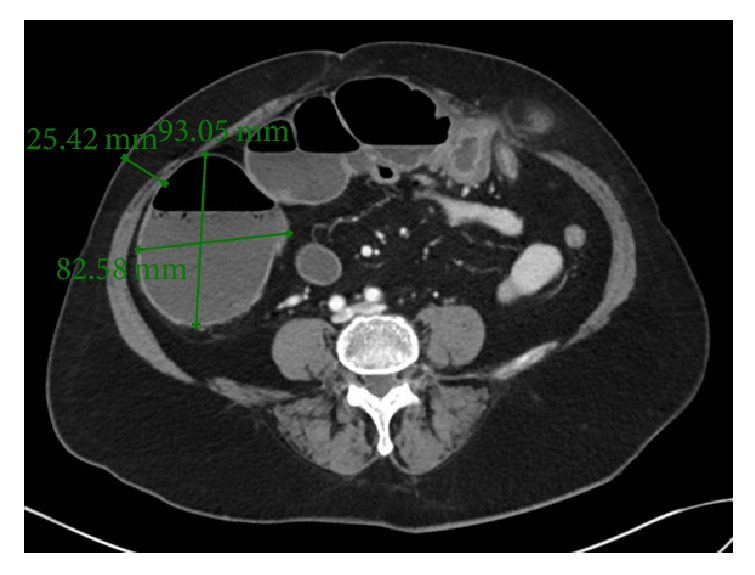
Axial CT showing significantly dilated caecum.
